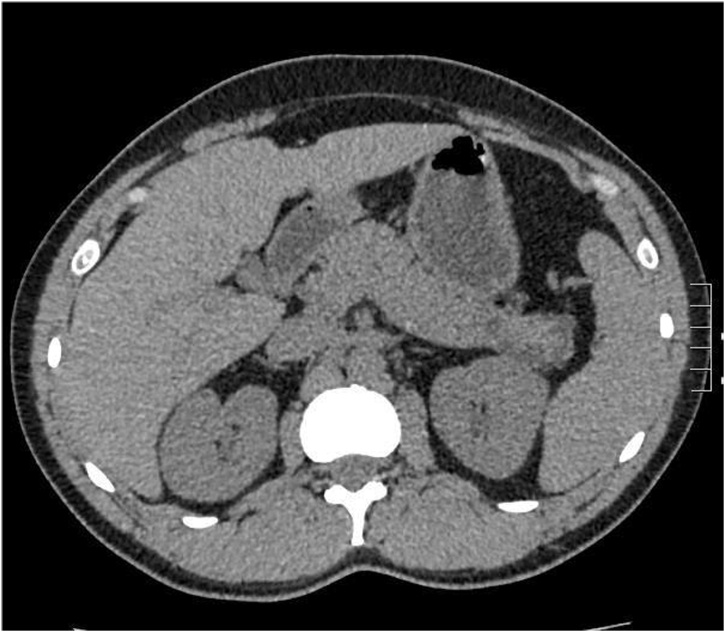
Non contrast CT scan of the abdomen of the shows few cystic lesions as the tail of pancreas.
